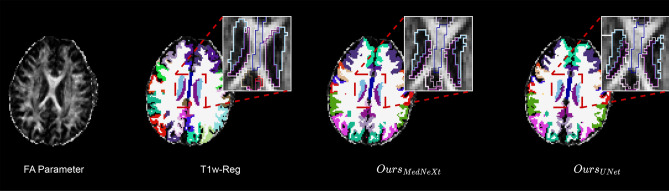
Parcellation comparison between our framework and the co-registered T1-weighted parcellation projected onto the dMRI space (T1w-reg) in the CNP dataset.
